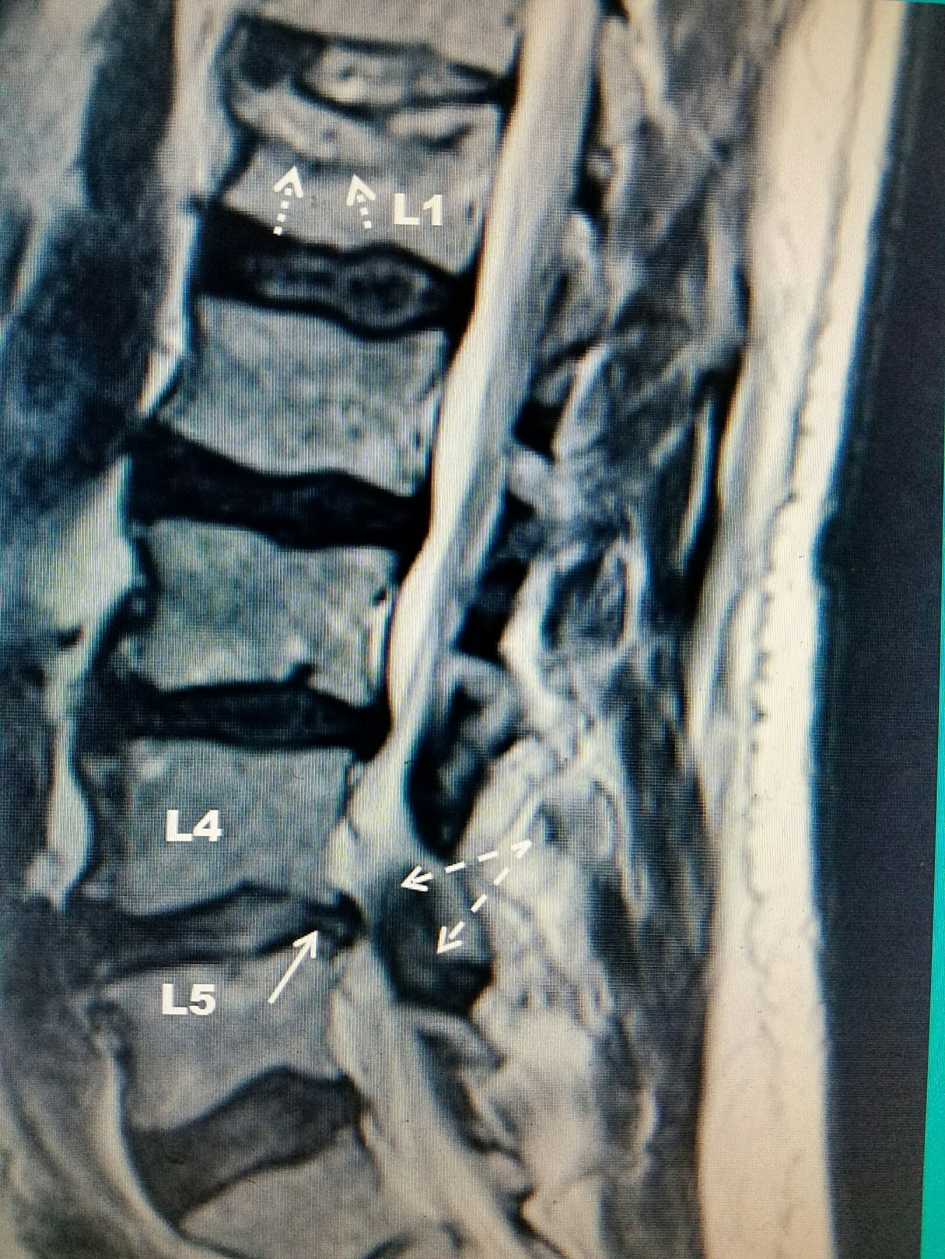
T2 weighted sagittal MRI demonstrating a L1 vertebral compression fracture in addition to L4/L5 central spinal stenosis
